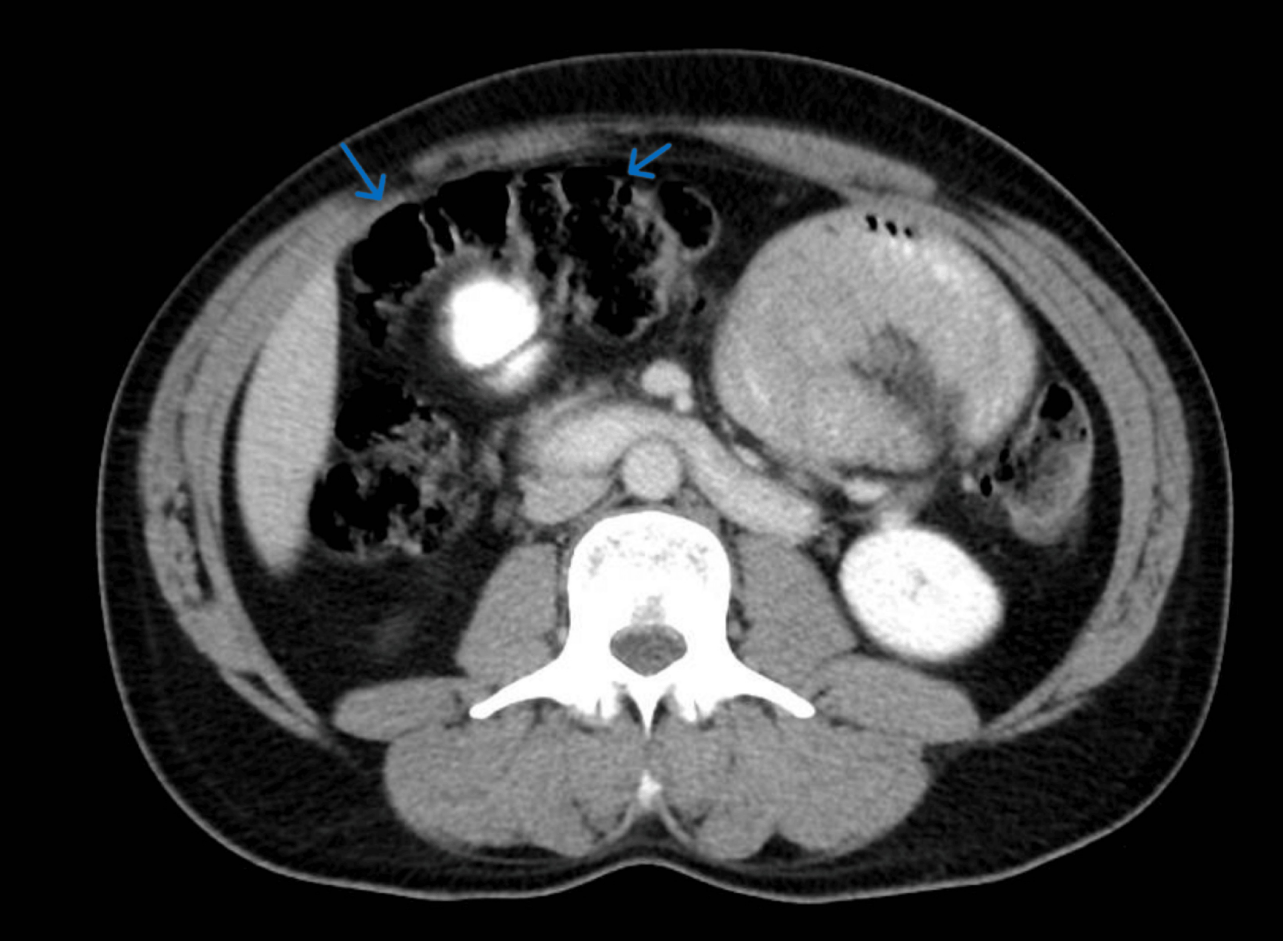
CT of the abdomen and pelvis with oral and IV contrast showing a clustering of small bowel loops likely encapsulated within a sac-like structure (blue arrows indicate small bowel loops) CT: computed tomography, IV: intravenous
